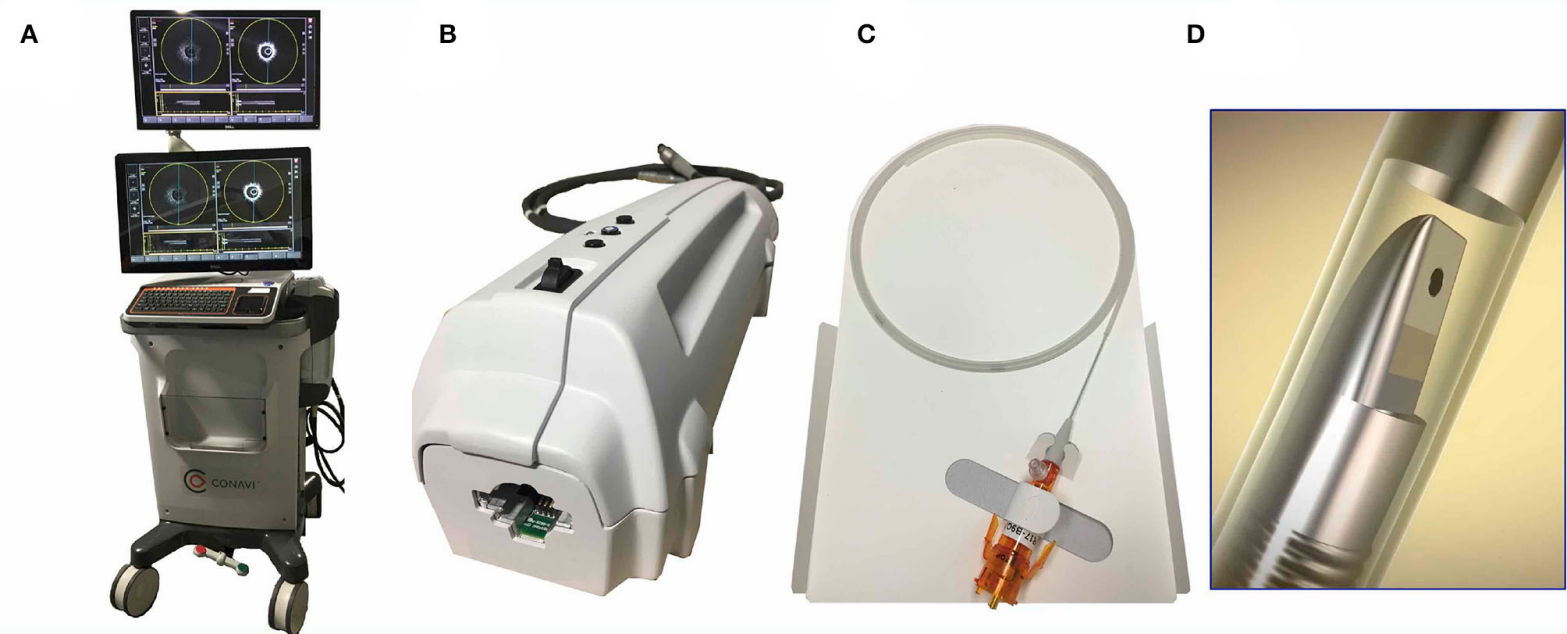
CONAVI Novasight hybrid imaging catheter; external appearances. (A) A whole appearance of system body. (B) Interface module. (C) Catheter technical specifications. (D) The transducer. IVUS, intravascular ultrasound; OCT, optical coherence tomography.
